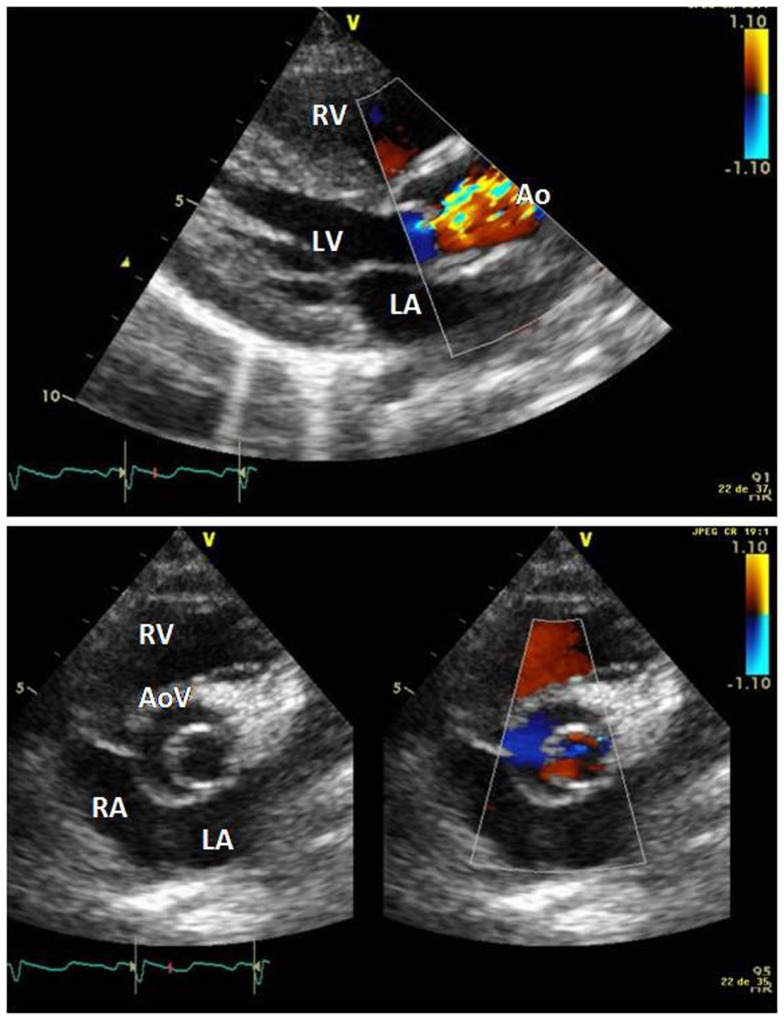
Long axis parasternal view showing doming of the aortic valve, aliaising of flow secondary to stenosis, and left ventricular hypertrophy. Short axis view showing bicuspid aortic valve. Abbreviations: AoV, aortic valve; LA, left atrium; LV, left ventricle; RV, right ventricle.
